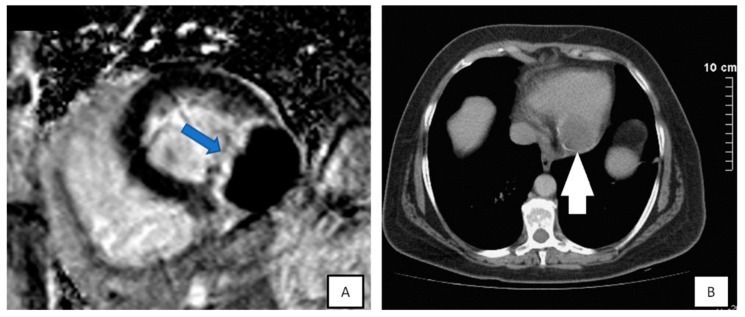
(A) Short-axis of late-gadolinium enhancement image demonstrating the echinococcus cyst attached to inferolateral wall, highly hypointense with hyperintense border (blue arrow). (B) CT demonstrated a calcified cyst (white arrow) proved to be a cardiac echinococcus cyst.
